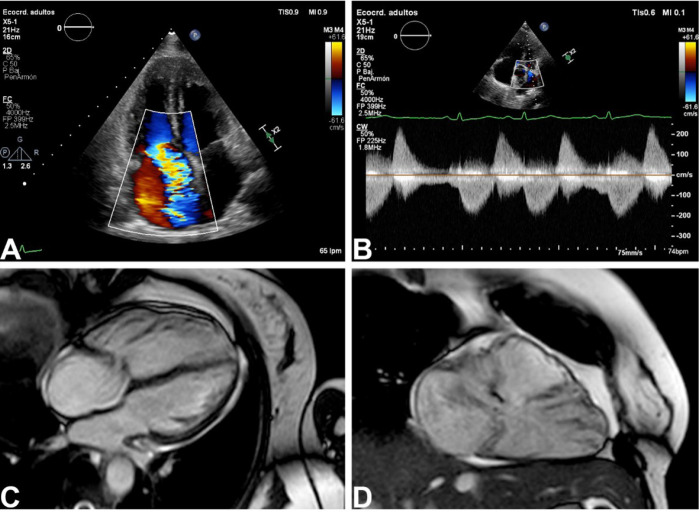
Echocardiography and cardiac magnetic resonance image (MRI) findings. On
